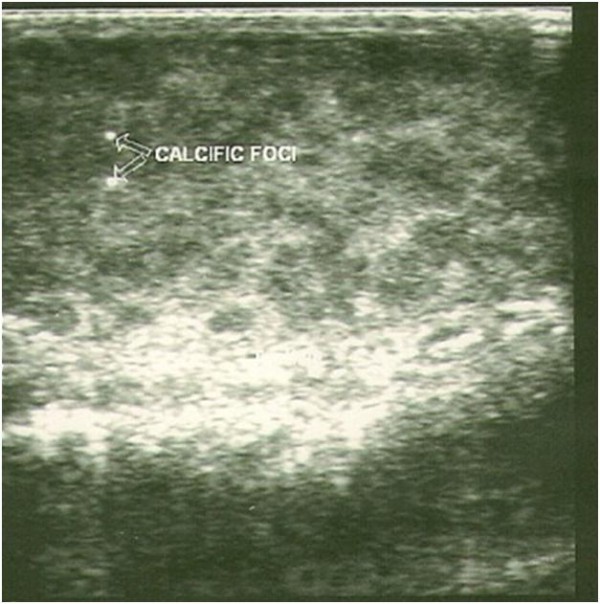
Ultrasound image showing enlarged left testis with diffuse hypoechoic masses and multiple foci of calcification within it.
